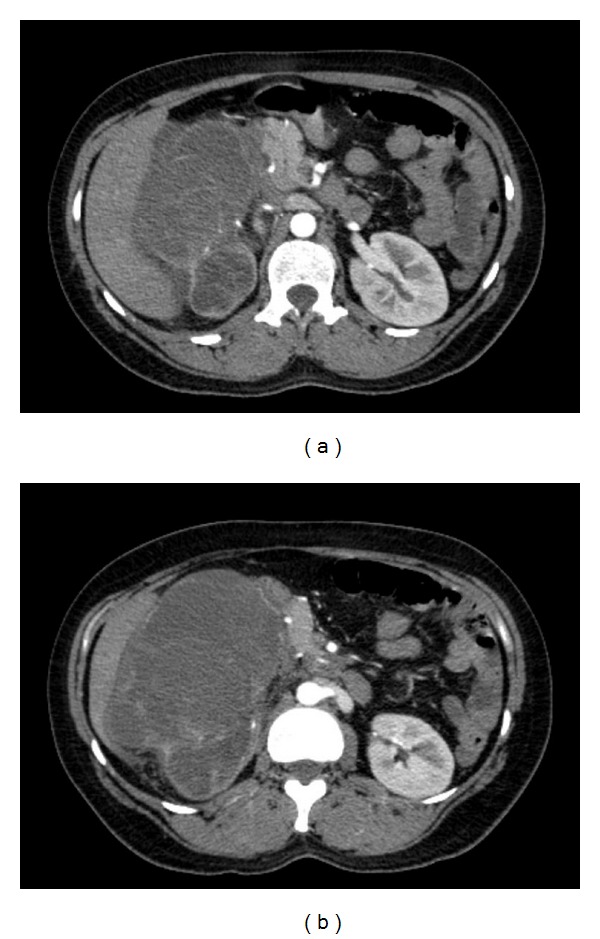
Cross-sectional computed tomography (CT) images showing a large heterogeneous mass replacing the right kidney.
